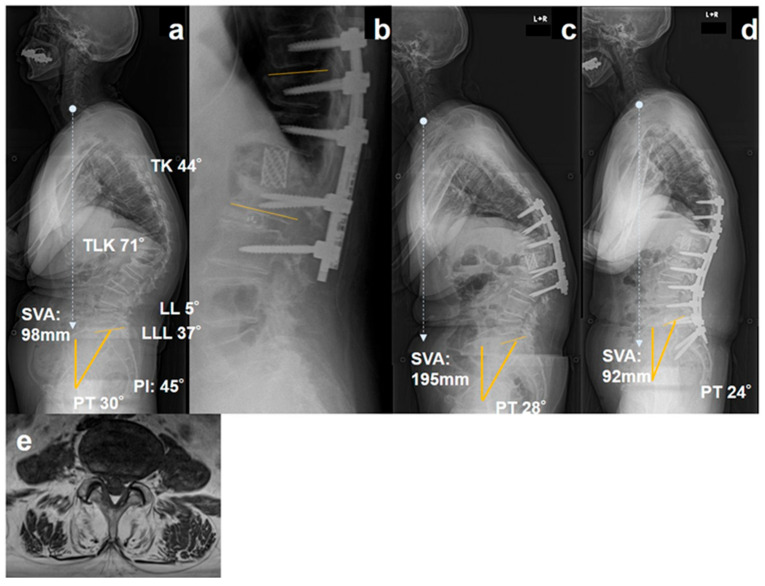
Distal junctional failure after short fixation without CAPS (Case 2). Preoperative standing lateral radiograph of a patient with an old OVFs from T10 to L2 showing moderate sagittal malalignment (SVA, 98 mm; PT, 30°; LL, 5°; LLL, 37°) (a). Postoperative lateral radiograph after posterior vertebral column resection and pedicle screw fixation showing acceptable local correction (b). Early distal junctional failure developed within 2 months after surgery with progressive collapse at the distal junction (c). Revision surgery with extension of fixation to the pelvis is required to restore sagittal balance (d). Axial T1-weighted MRI at the L4–L5 shows paraspinal muscle fatty degeneration corresponding to Goutallier grade 3 (e).
